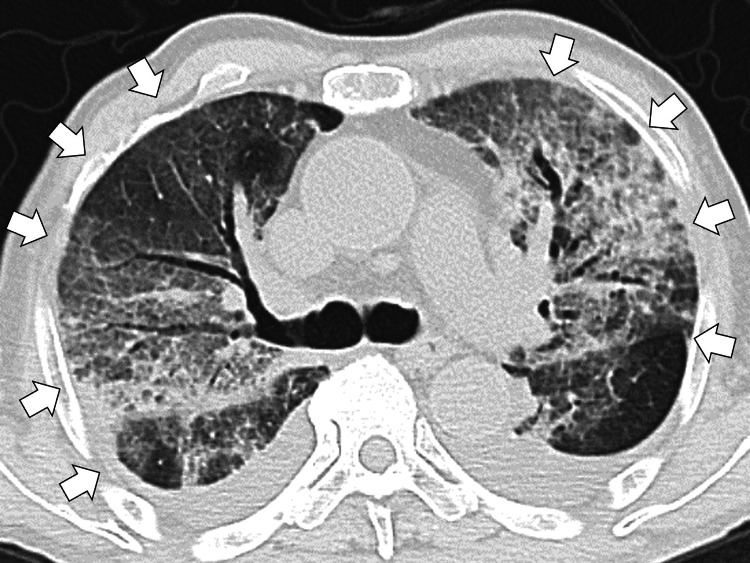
Computed tomography of the chest showing diffuse ground-glass opacities in both lungs (white arrows)
